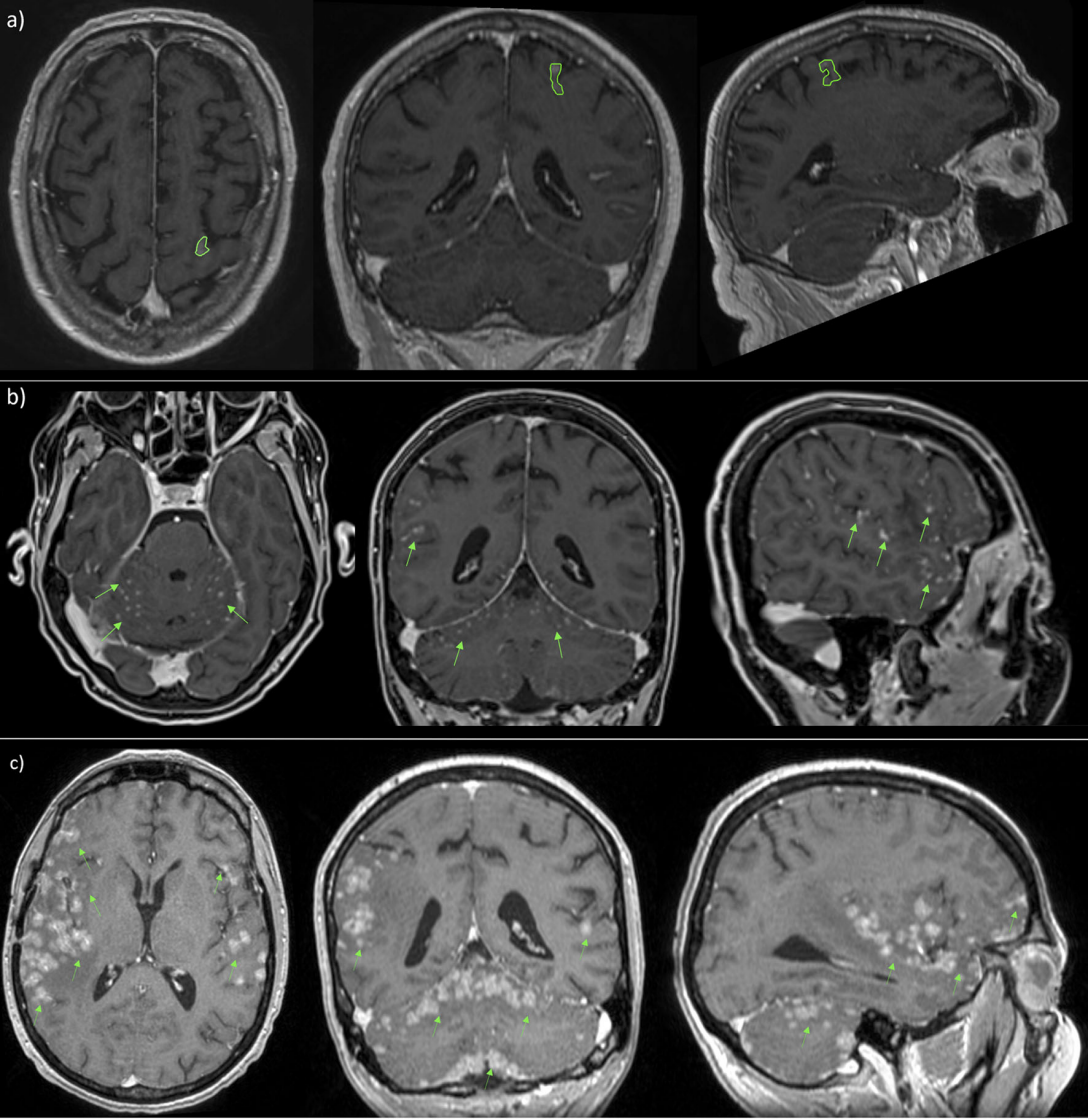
MRI scans in patient with nodular (A), linear (B) and both types (C) of leptomeningeal metastasis.
